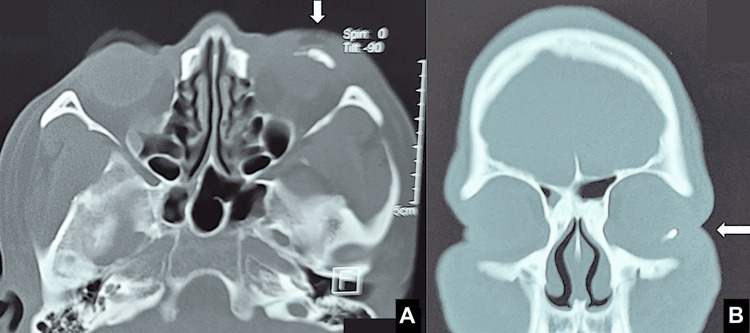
Axial (A) and coronal (B) CT images of the head and orbits. Arrows indicate the molten aluminum cast on the left eye
